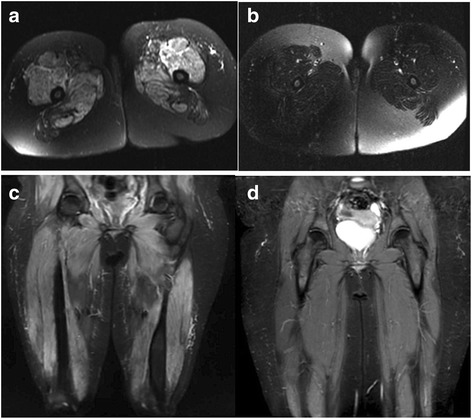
MRI at time of diagnosis and flares. a and c showed the axial and coronal section of the first MRI at the time of diagnosis. It showed diffusely increased T2 signal throughout all the muscles of the thigh as well as of the pelvis and hip girdle region with symmetrical involvement except for more patchy involvement in the adductors and in semi-membranous and semi-tendinous muscles. b and d showed the axial and coronal section of the second MRI at the time of flare. It showed: bilateral increased intramuscular T2 signal within both posterior calves including the lateral head of the gastrocnemius, soleus, and medial head of gastrocnemius and plantaris muscles with corresponding areas of restricted diffusion. The degree of restricted diffusion and T2 prolongation has markedly decreased compared with initial MRI A and C. There is subtle T2 prolongation with corresponding restricted diffusion diffusely within the anterior thigh musculature involving the bilateral vastus lateralis, rectus femoris and subtly within the vastus medialis muscles
